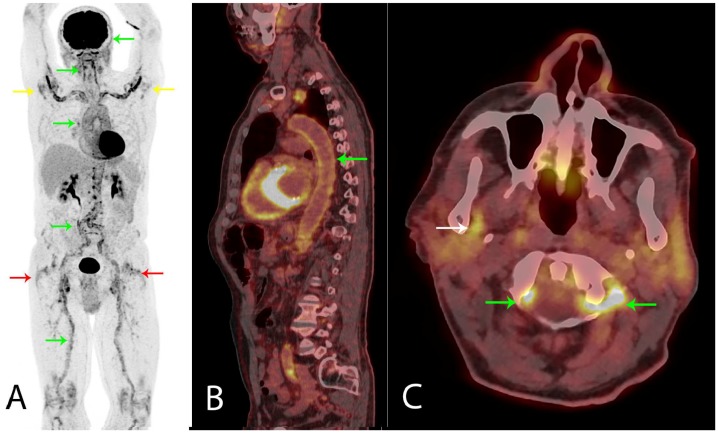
A healthy 80-year old man had, during the last six month period, experienced a 5 kg non-intended weight loss, malaise, coughing, and extreme fatigue. Before the symptoms started, he was physically active and bicycled 20–40 km and rowed 10–15 km per week. As symptoms progressed, muscle tenderness in the shoulder and hip regions made exercising impossible. No morning stiffness of the joints was experienced. Blood tests showed a mild normocytic normochromic anemia, elevated C-reactive protein (100 mg/L), and erythrocyte sedimentation rate (88 mm/h). PMR was suspected, but malignancy was a differential diagnosis. A total of 3.85 MBq/kg ^18^F-FDG was administrated an hour before a whole body PET/CT scan was performed. (A) Frontal PET MIP showed highly increased ^18^F-FDG uptake diffusely around shoulder (yellow arrows) and hip joints (red arrows), indicating bursitis, but also in the wall of the large arteries (green arrows), indicating synchronic LVV confirmed on (B) sagittal fused PET/CT of the aorta (green arrow). The LVV aorta and its major branches are affected, but the vertebral (green arrows) and maxillary (white arrow, right side shown) arteries could also be involved, (C) axial fused PET/CT. Notably, the ^18^F-FDG uptake in the affected arteries (not quantitatively shown) was more than two times the uptake in the liver, which has been shown to accurately indicate LVV on PET/CT [1,2,3,4].
